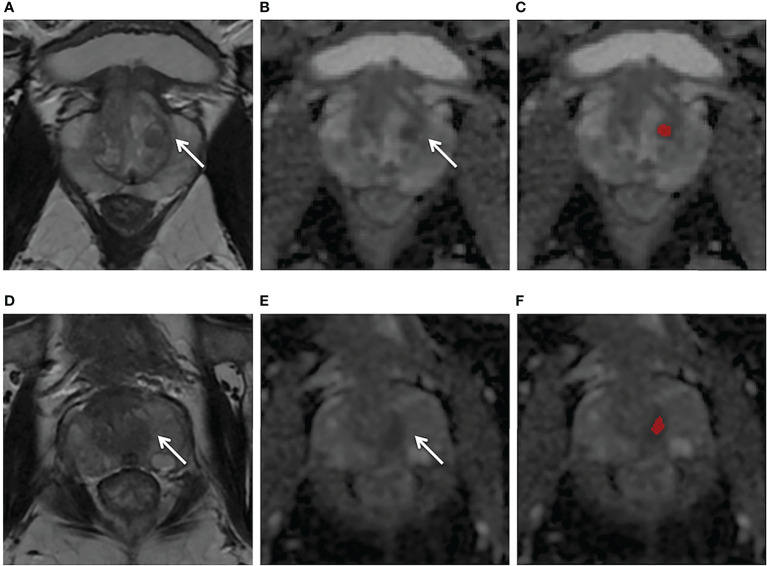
(A–C) Axial MR images obtained in a 56-year-old patient with a PSA level of 4.2 ng/ml and with negative biopsy findings. T2WI (A) showed a heterogeneous encapsulated nodule in the left transition zone (arrow) and the ADC map (B) showed hypointensity (arrow). The ADC model (C) appeared false positive (red region). (D–F) Axial MR images obtained in a 64-year-old patient with a PSA level of 5.9 ng/ml and with negative biopsy findings. T2W (D) and ADC (E) showed a normal left central zone, while the ADC model (F) appeared false positive in this area (red mark). The biparametric model gave negative predictive values for both cases.
